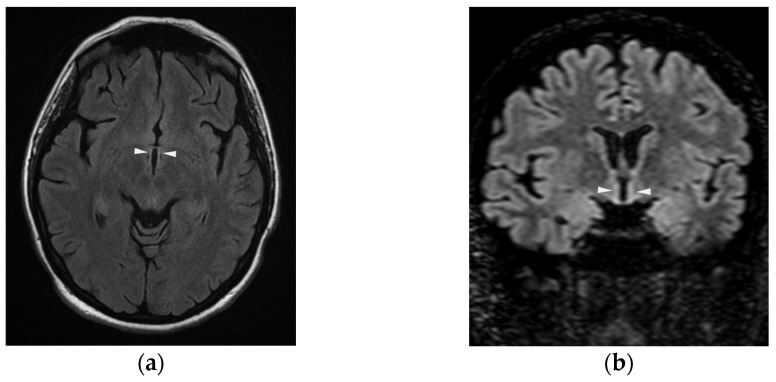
FLAIR views of periventricular region of the third ventricle in axial (a) and coronal (b) perspectives.
